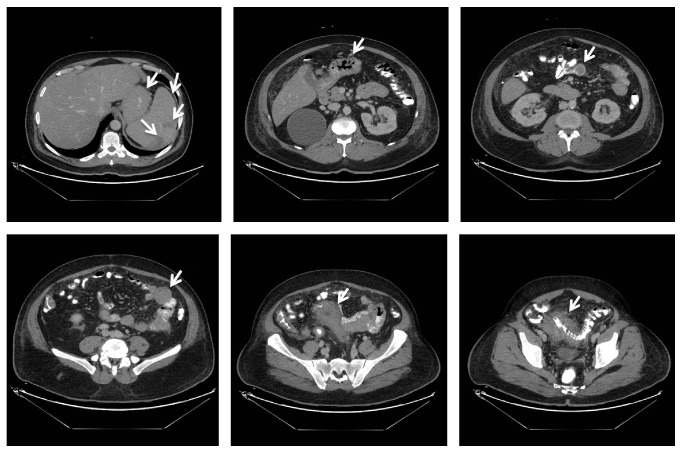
CT NCAP demonstrating a large soft tissue mass surrounding the sigmoid with mild enhancement measuring around 9.2 × 12 × 6 cm, multiple enhanced soft tissue masses surrounding multiple segments of the small bowel, multiple peritoneal implants, and multiple mesenteric subcentimetric lymph node enlargement. There were also circumferential stomach wall thickening measuring around 2.8 cm, small subcentimetric left axillary and periaortic lymph nodes, splenomegaly, and two splenic enhancing lesions.
